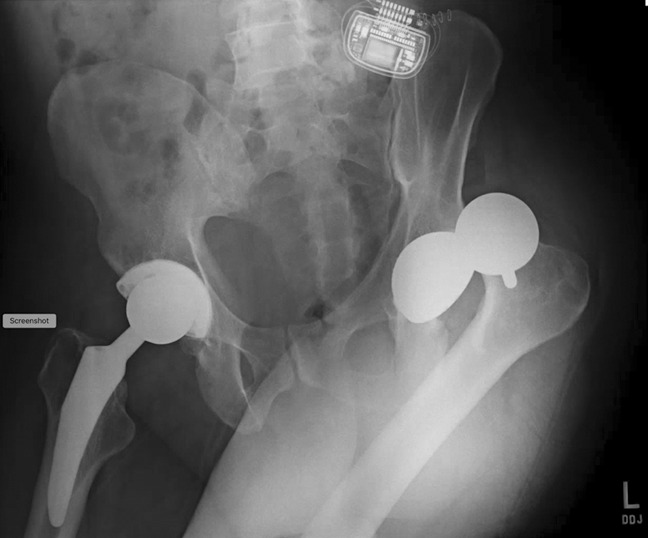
Radiograph revealing dislocation of a well-fixed and functioning Birmingham hip resurfacing immediately after surgical placement of a spinal cord stimulator for pain management of back pain.
